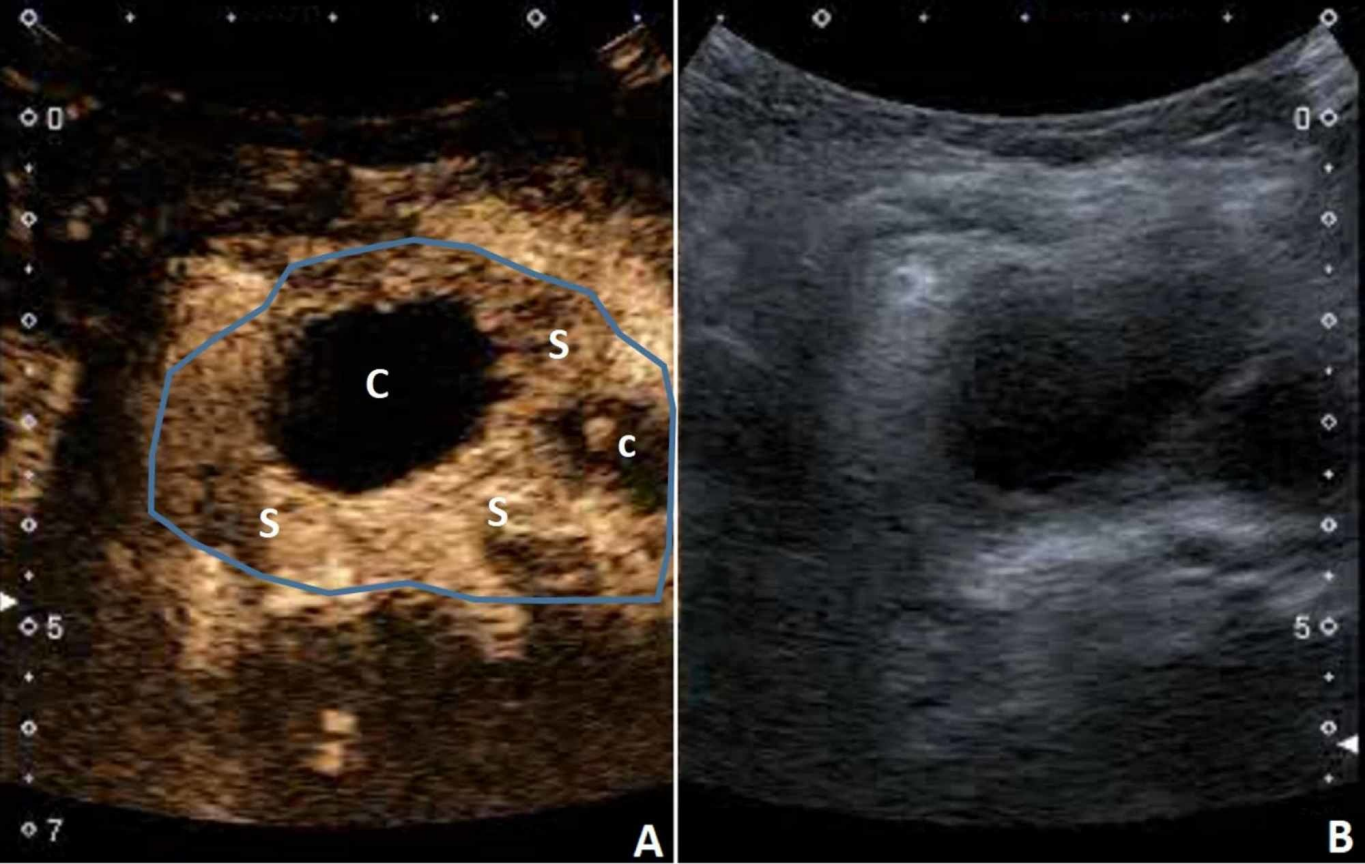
CEUS demonstrated peripheral enhancement of the mass (blue outline). Cystic parts (C) and solid parts (S) are clearly demarcated. (B) B-mode US of the neck mass. CEUS, contrast-enhanced ultrasound US, ultrasound
